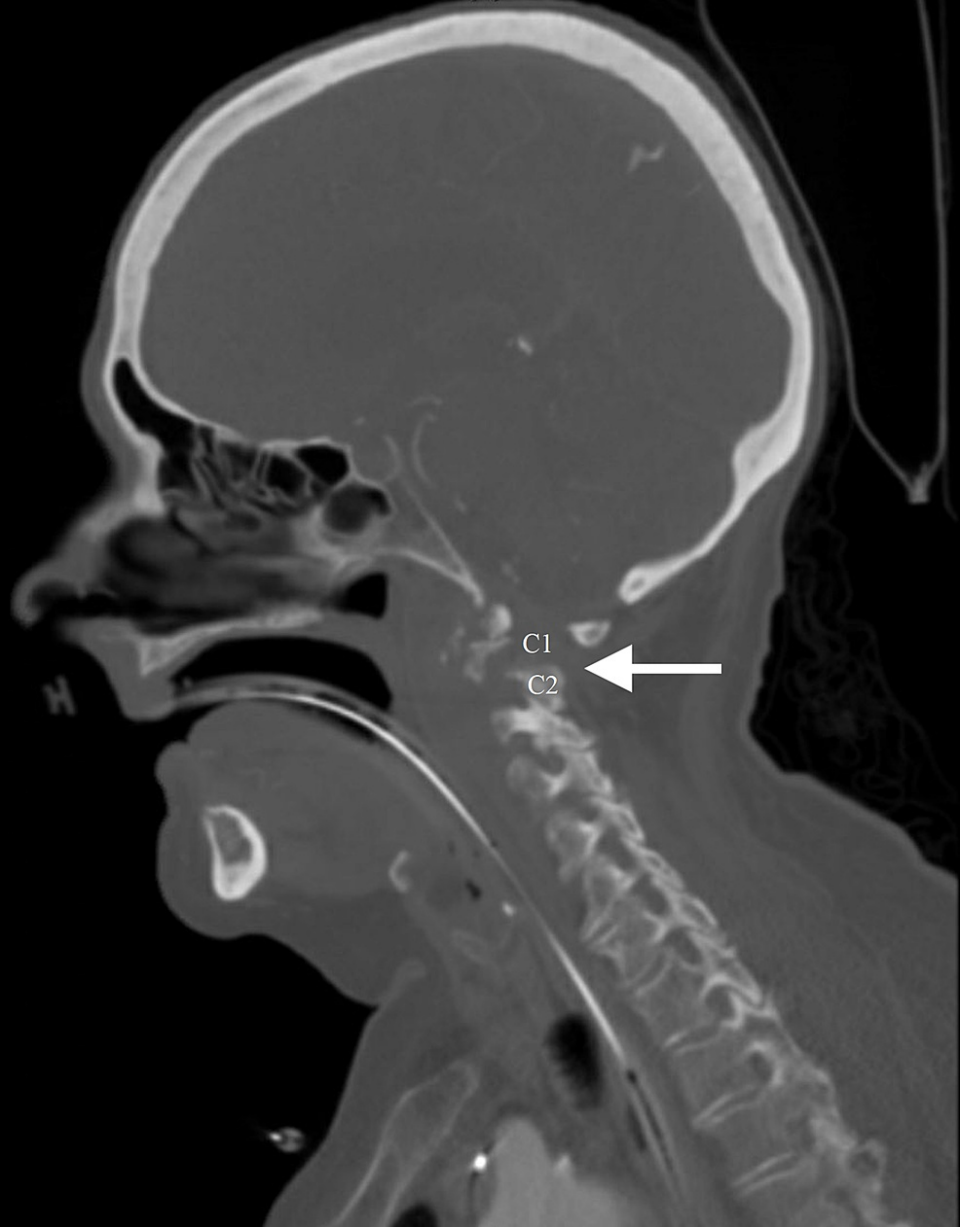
Cervical spine CT scan reveals C1-C2 degeneration with loss of bone integrity (arrow). CT: computed tomography C1: cervical vertebra one C2: cervical vertebra two
